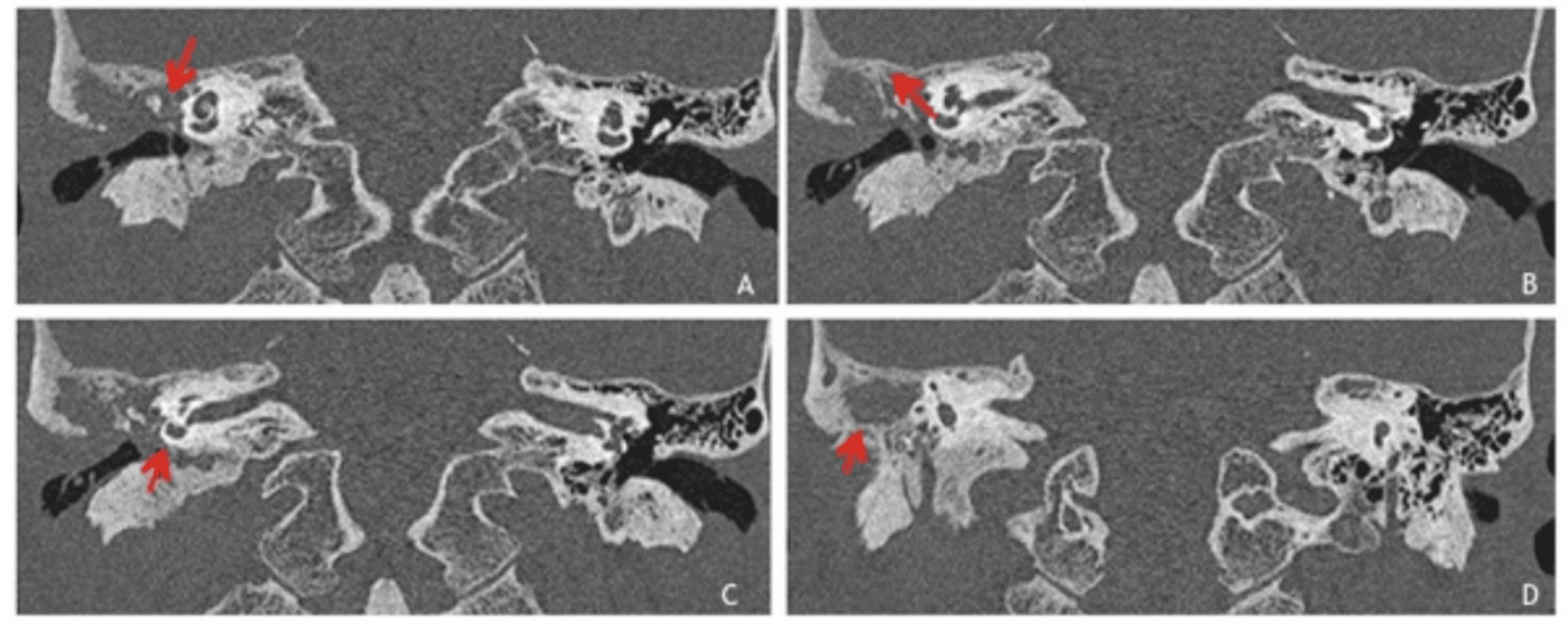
HRCT temporal coronal images showing soft tissue density lesion in middle ear cavity (in epitympanum, mesotympanum and hypotympanum, and Prussack’s space) and mastoid air cells with the destruction of the mastoid septae, outer mastoid cortex and adjoining bony external auditory canal and causing thinning and erosions of tegmen tympani. HRCT: High-resolution computed tomography
